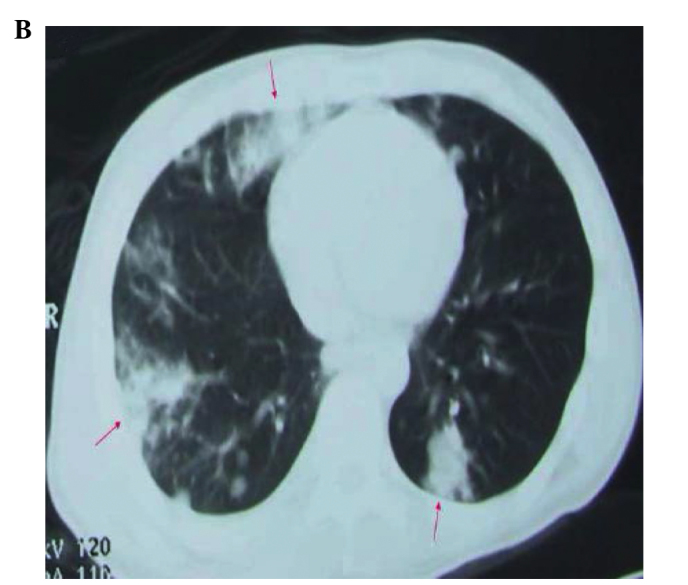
(A) Gadolinium contrast-enhanced magnetic resonance imaging shows a large tumor at the sacrum, with compression of the intestine and urinary bladder. The pink arrows indicate the tumor edge and the asterisk indicates the center of the tumor. (B) Multiple metastases in the bilateral lung fields. The pink arrows indicate the the tumor edge.
